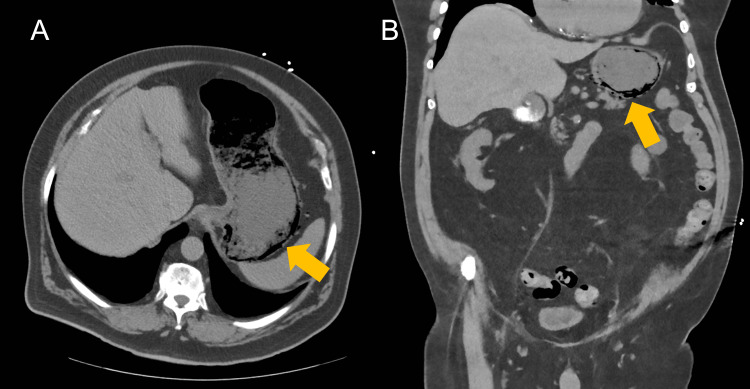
(A) Axial and (B) coronal CT abdomen images demonstrating gastric emphysema of the posterior wall and fundus of the stomach. The arrows indicate the areas demonstrating gastric emphysema. CT: computed tomography
